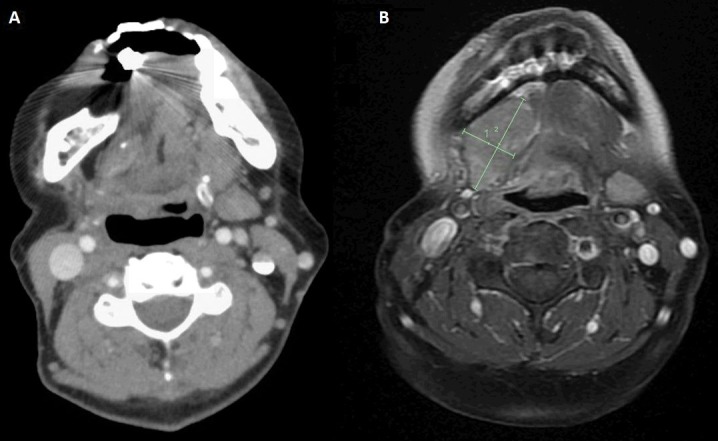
Diagnostic imaging (A) Computerized tomography (CT) scan of the neck with contrast performed in May 2016. Postoperative changes of right-sided partial maxillectomy and marginal mandibulectomy with flap reconstruction can be seen. There was a tissue with intermediate density in the infratemporal fossa, and partially surrounding the right pterygoid process. At the time of the exam, the radiologist could not conclude whether this was scar tissue or tumor recurrence. (B) Magnetic resonance imaging (MRI) scan of the neck with contrast performed in June 2016. The lesion was seen along the right side of the floor of the mouth measuring 1.9 x 3.5 x 3.9 cm in maximum dimensions compared to 1.4 x 2.5 x 2.3 cm along the same dimensions previously. The lesion was inseparable from the right mylohyoid muscle and of the remainder of the floor of the mouth muscles. No definite invasion or significant abnormality of the adjacent portion of the mandible was seen.
